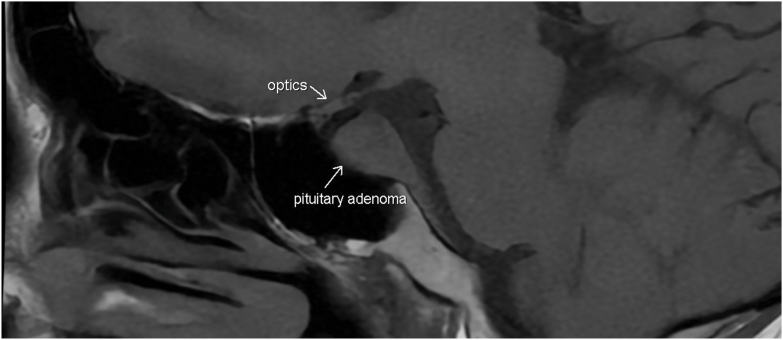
MRI pituitary sagittal image revealing 1.8 × 1.9 cm sellar mass.
